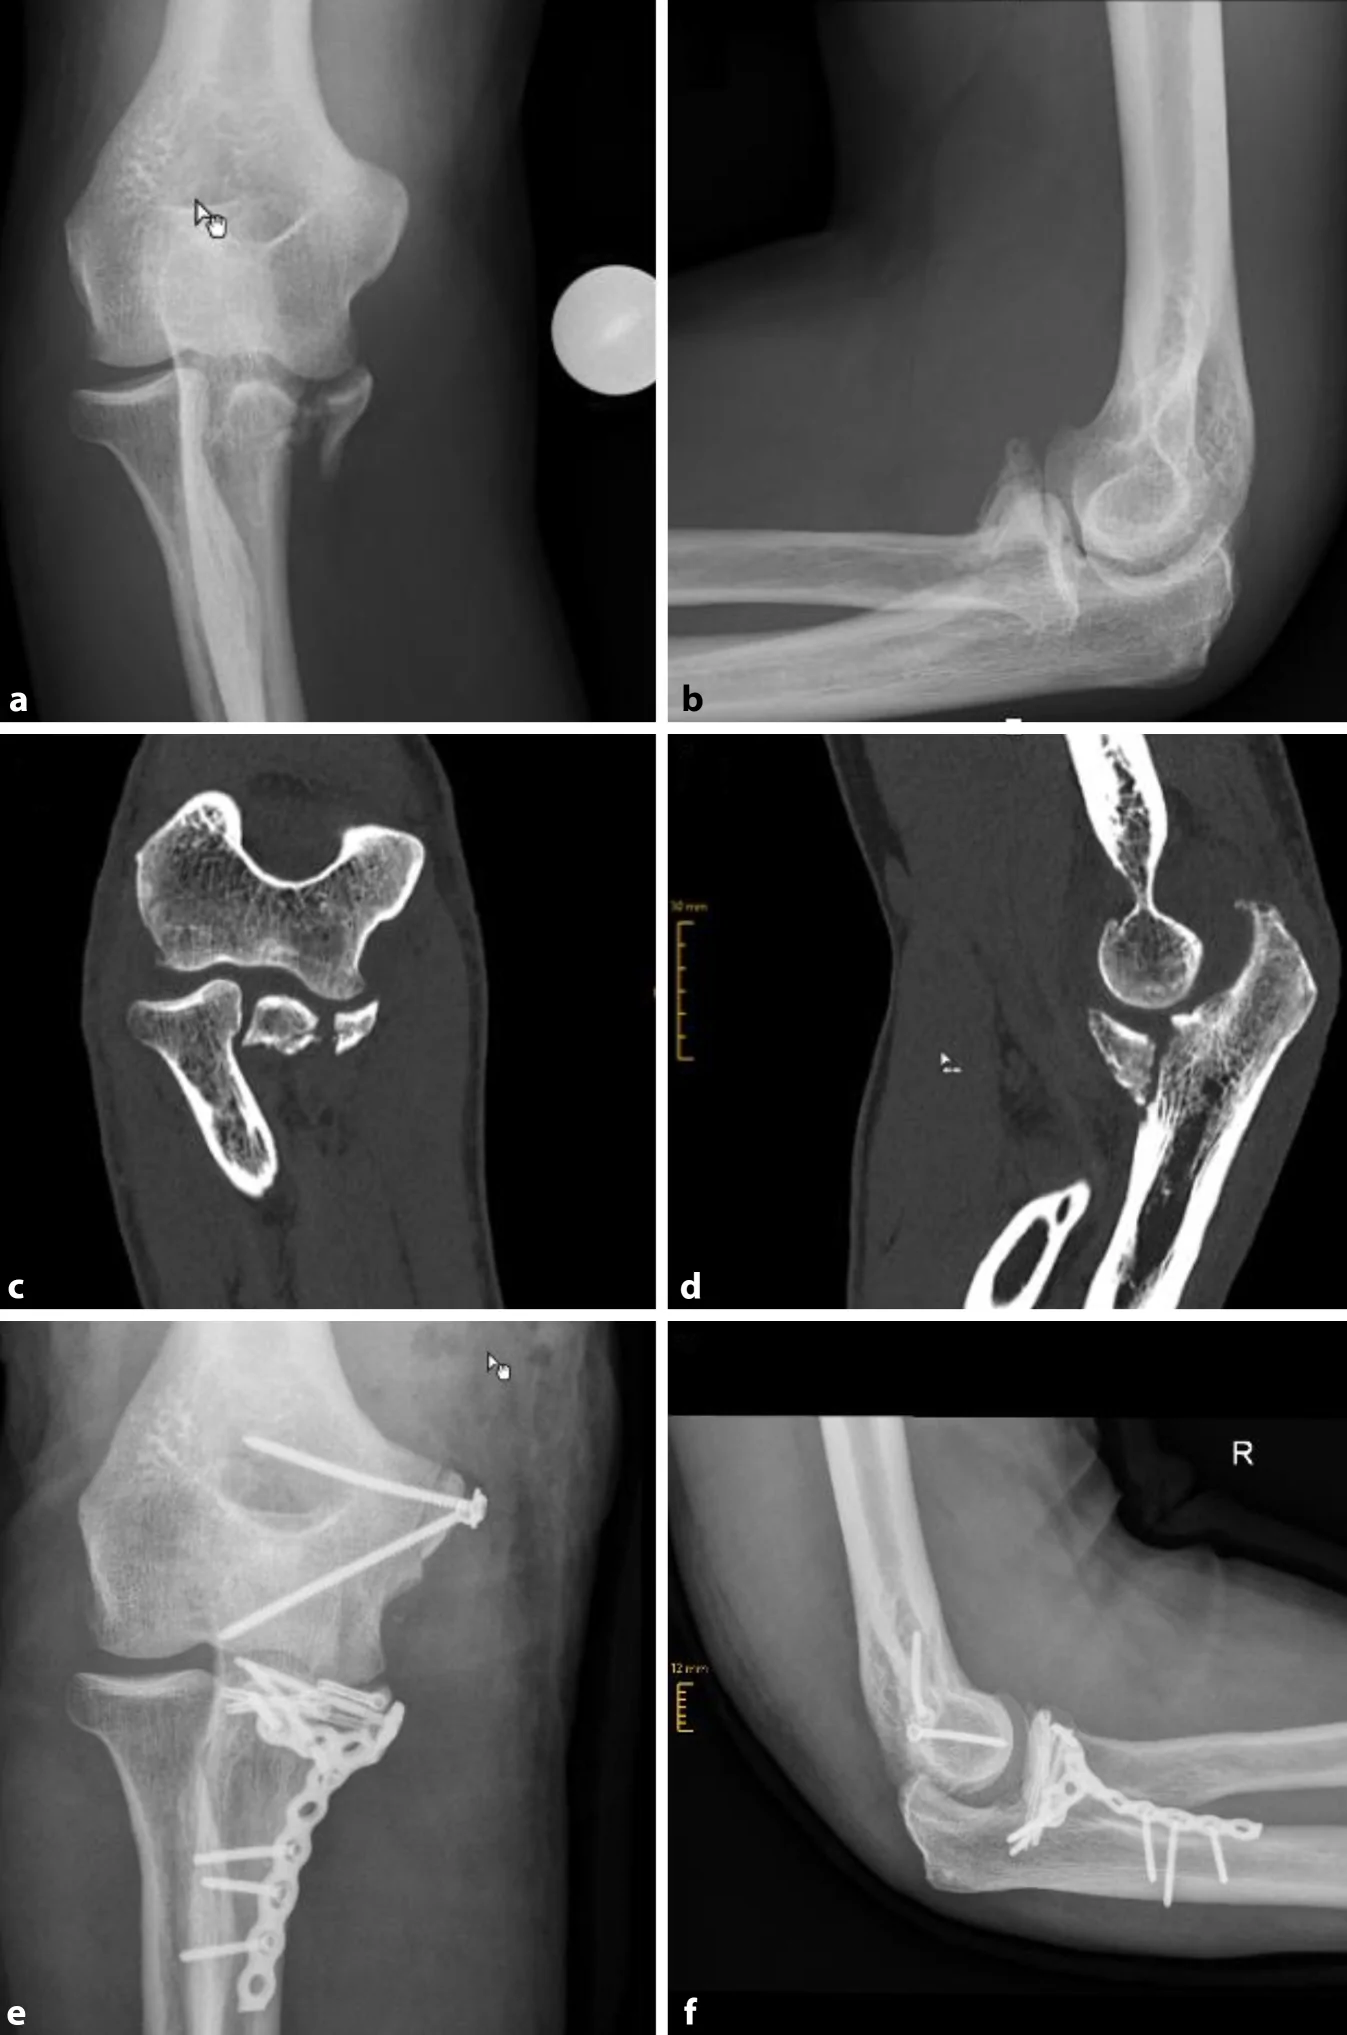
Radiographs (a, b) and computed tomography (c, d) demonstrate an O’Driscoll anteromedial type 3 fracture of the anteromedial facet and tubercle. Due to a delayed presentation, the patient underwent buttress plate fixation through a medial epicondyle osteotomy 7 weeks after trauma (e, f)
